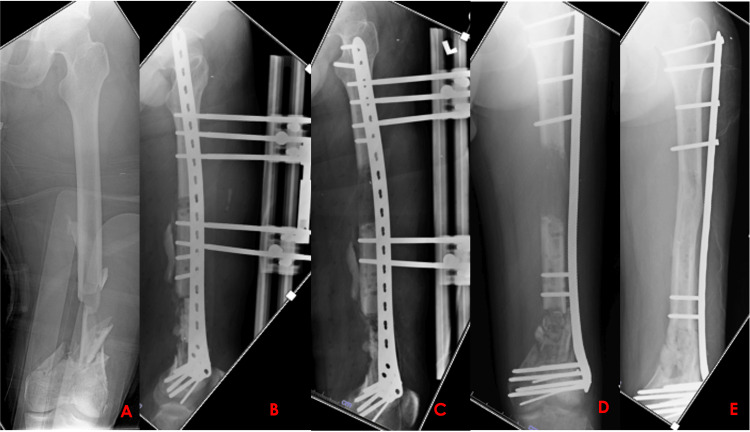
Serial radiographs of a case of acute shortening, followed by bone lengthening over plate + induced membrane technique (IMT). (A) Initial trauma film depicting injury with bone loss of 13 cm. (B) The defect is shortened by half, followed by fixation with a locking plate and application of monolateral rail and proximal corticotomy for lengthening. (C) Proximal locking screws are inserted after completion of lengthening. (D) Autologous bone graft performed for the distal IMT segment. (E) Radiograph at nine months showing fracture site union and transport segment consolidation.
